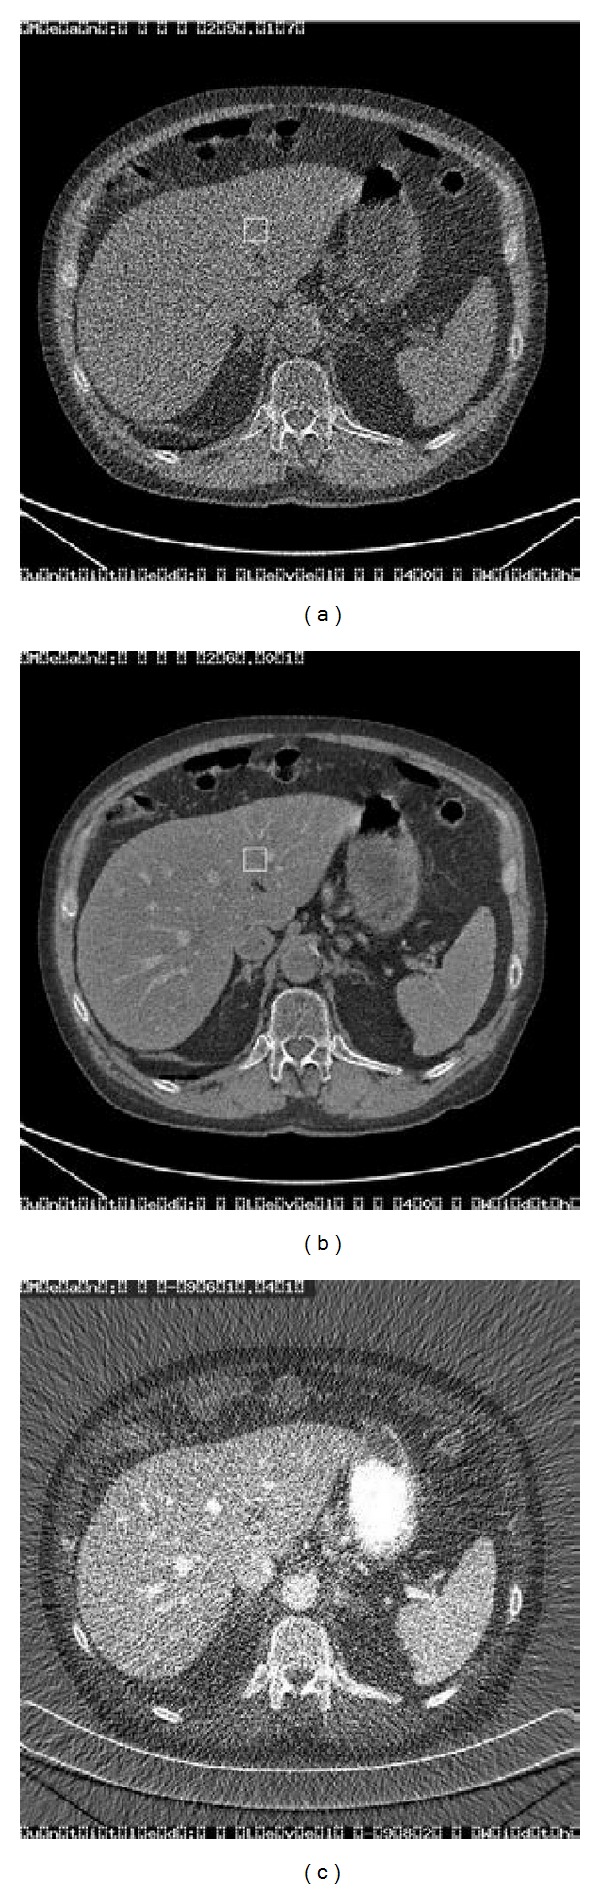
Comparison of the original and noise-reduced basis material (water) density images from a dual-energy abdominal CT exam of a patient. (a) Original water density image. (b) Noise-reduced water density image using the ACNR algorithm [12]. In spite of the fact that the noise in the image is reduced, artifacts are evident throughout the liver area. (c) Original iodine density image. The iodinated hepatic vessels correlate well with the artifacts in the water density image, suggesting that the artifacts are caused by cross-contamination.
